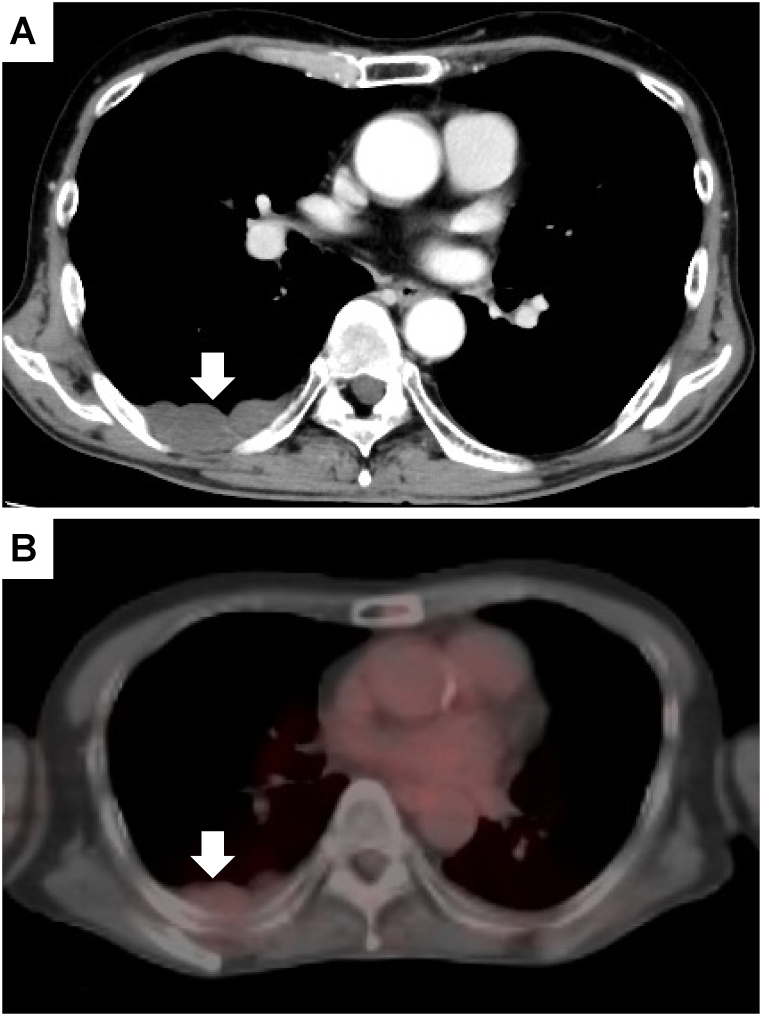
Contrast-enhanced computed tomography (CT) scan reveals a hypoattenuating lesion on the patient's right dorsal pleura (A), which shows no fluorodeoxyglucose uptake on positron emission tomography CT (B). The white arrow indicates the lesion.
